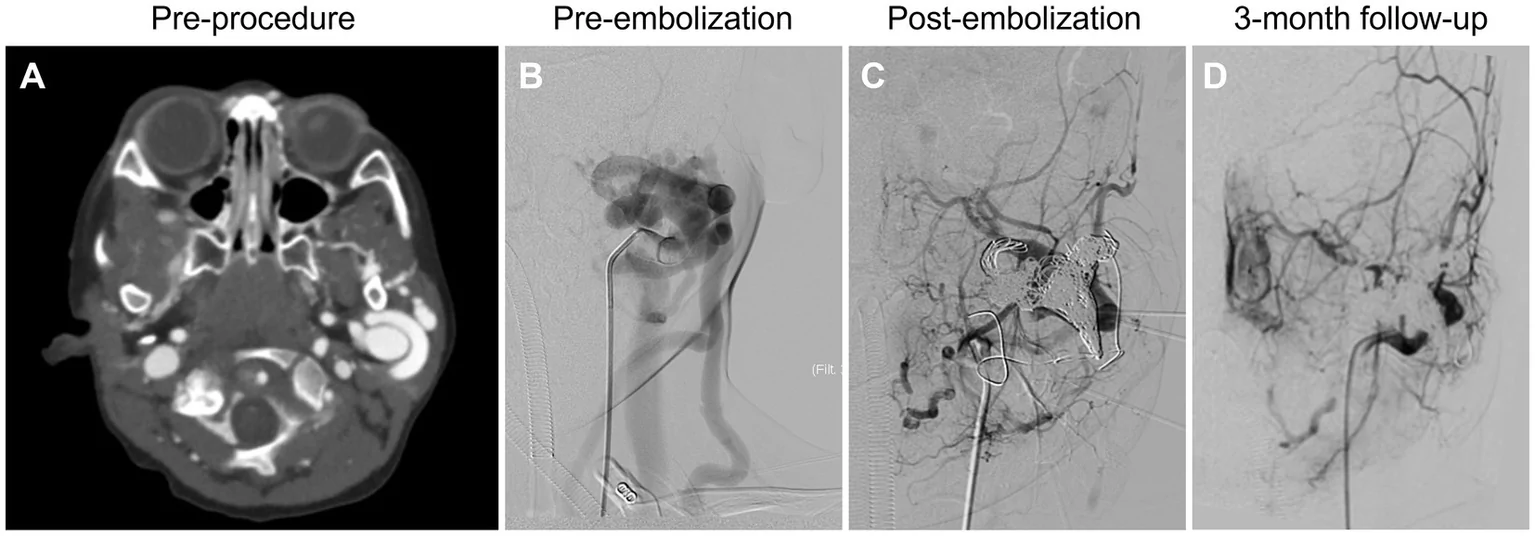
Endovascular embolization of a left parotid arteriovenous fistula in a 3-year-old boy (Patient # 2). (A) Preprocedural contrast-enhanced computed tomography revealed dilated, enhanced vessels in the left parotid region. (B) Digital subtraction angiography confirmed the fistula with a tortuous feeding artery and early venous opacification. (C) Post-embolization angiography showed complete occlusion of the fistula with opacification of distal arterial branches, indicating improved distal perfusion. (D) Angiography at 3-month follow-up demonstrated no recurrence of the fistula.
